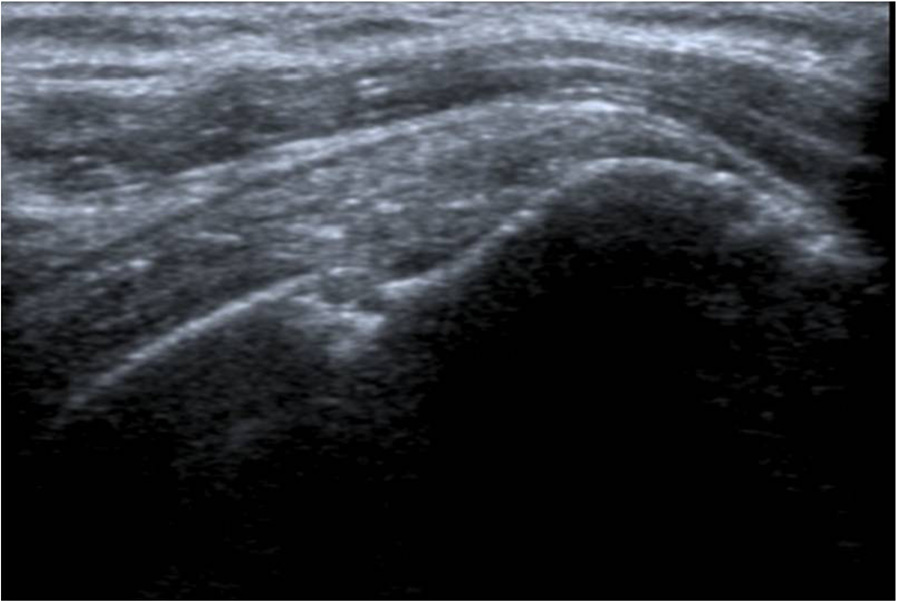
Ultrasound image of supraspinatus enthesitis: sagittal B-mode US scanning of supraspinatus tendon showing bony erosion with enthesophytes.
